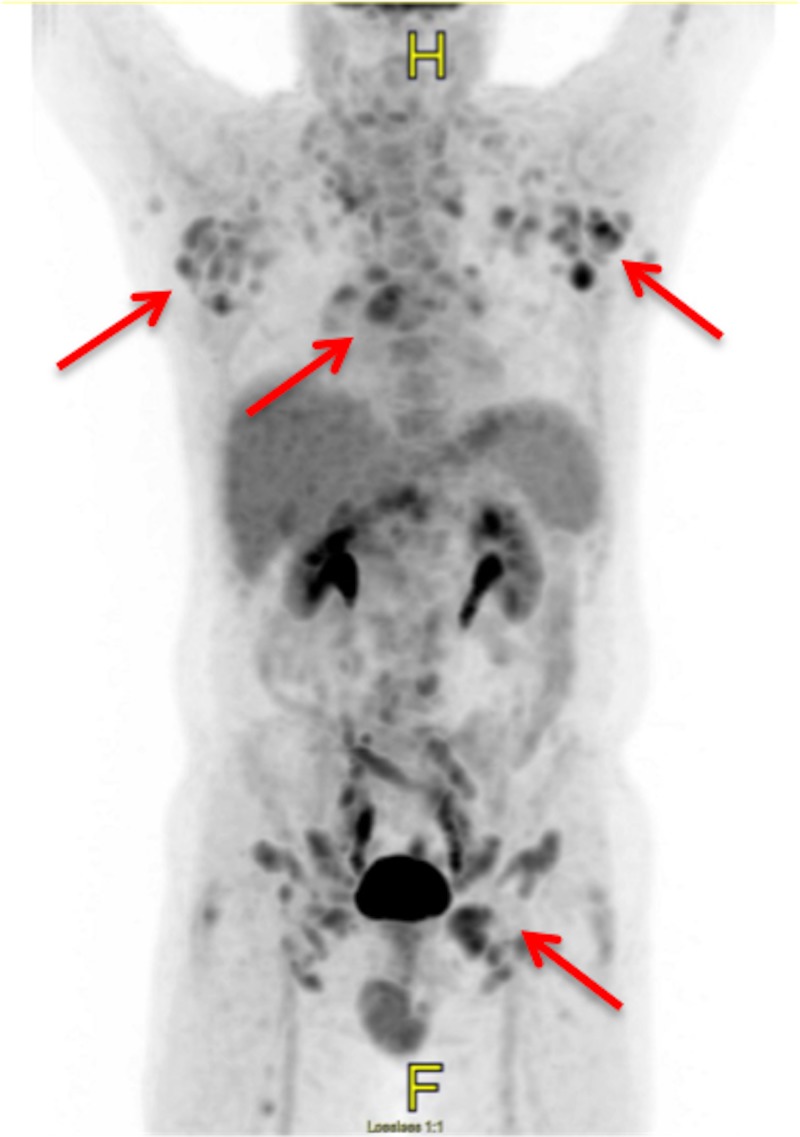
Initial PET/CT PET/CT scan at diagnosis showing extensive metabolically active lymphadenopathy (arrows). PET/CT: positron emission tomography/computed tomography
